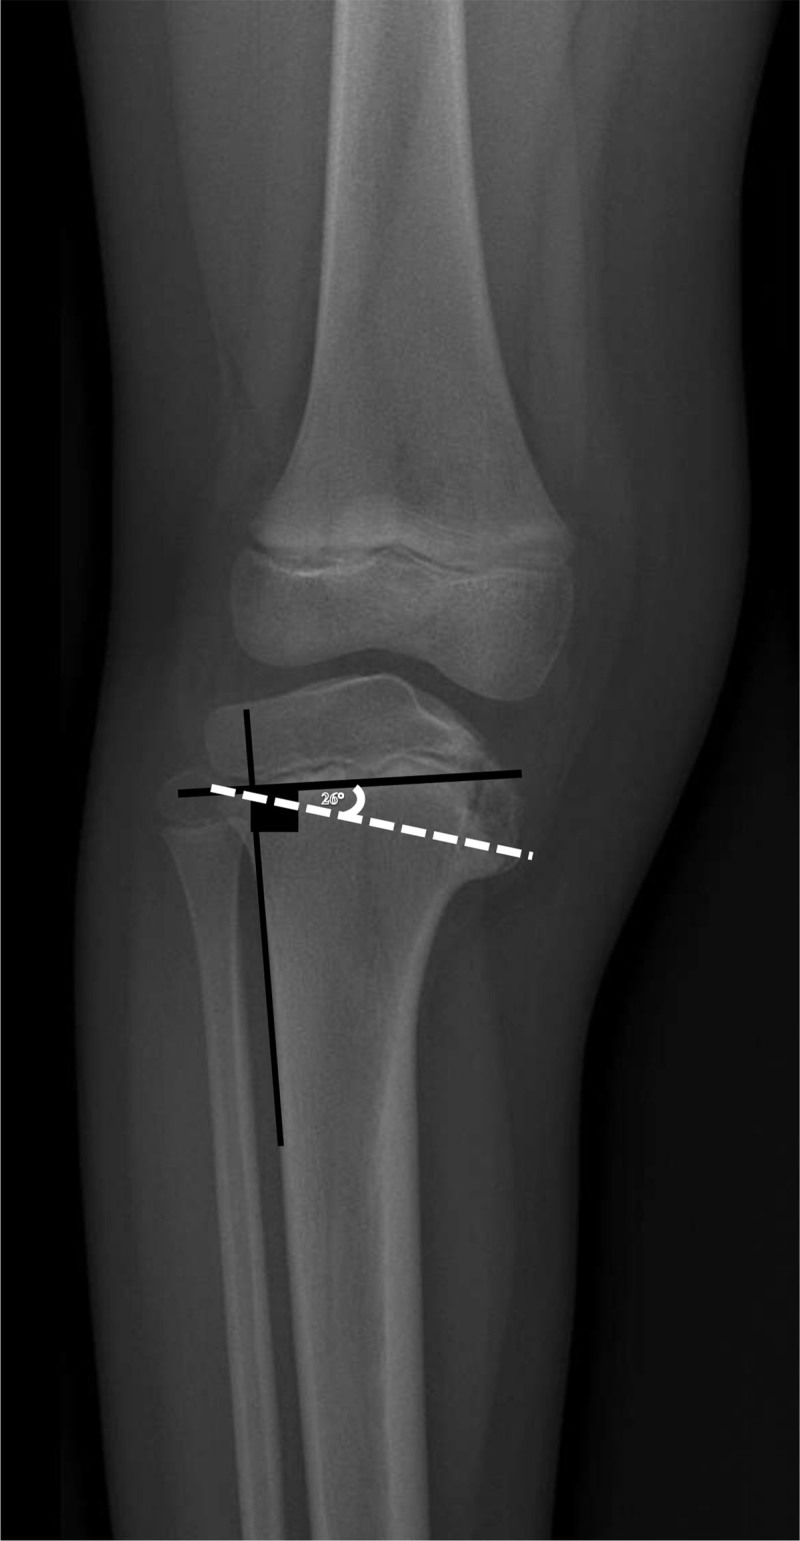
Besides the visualization of cartilage and menisci, MRI allows a three-dimensional assessment of the deformity. Some studies suggested that dynamic contrast-enhanced MRI could detect irreversible alterations of the physis. Untreated Blount disease is associated with a poor prognosis, especially in young children due to the growth spurt and the early fusion of the growth plate occurring from the ages of six to eight [1].
